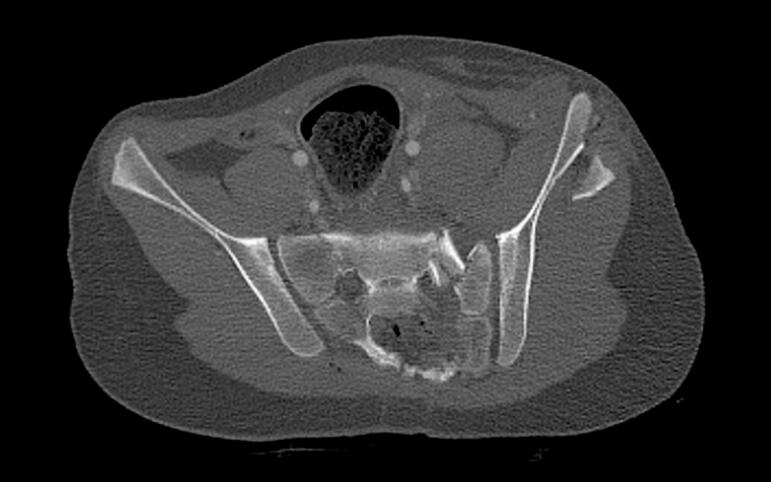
Transversal view of CT-scan of a 12 year old female who sustained a Tile type B3 Pelvic fracture.
